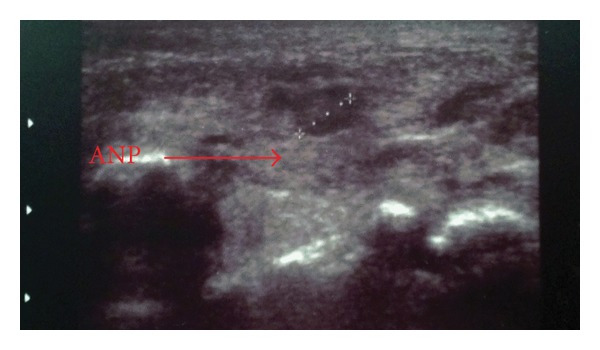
Sonogram of left acute neonatal parotitis (ANP).
